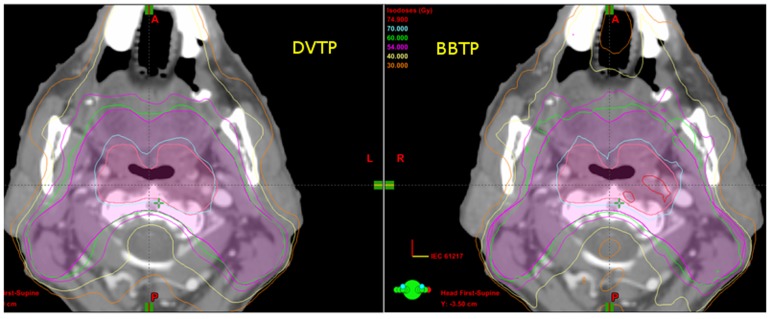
One of the axial computed tomography (CT) slices comparing the isodose curves between the BBTP plan and the DVTP plan of a typical NPC patient.
